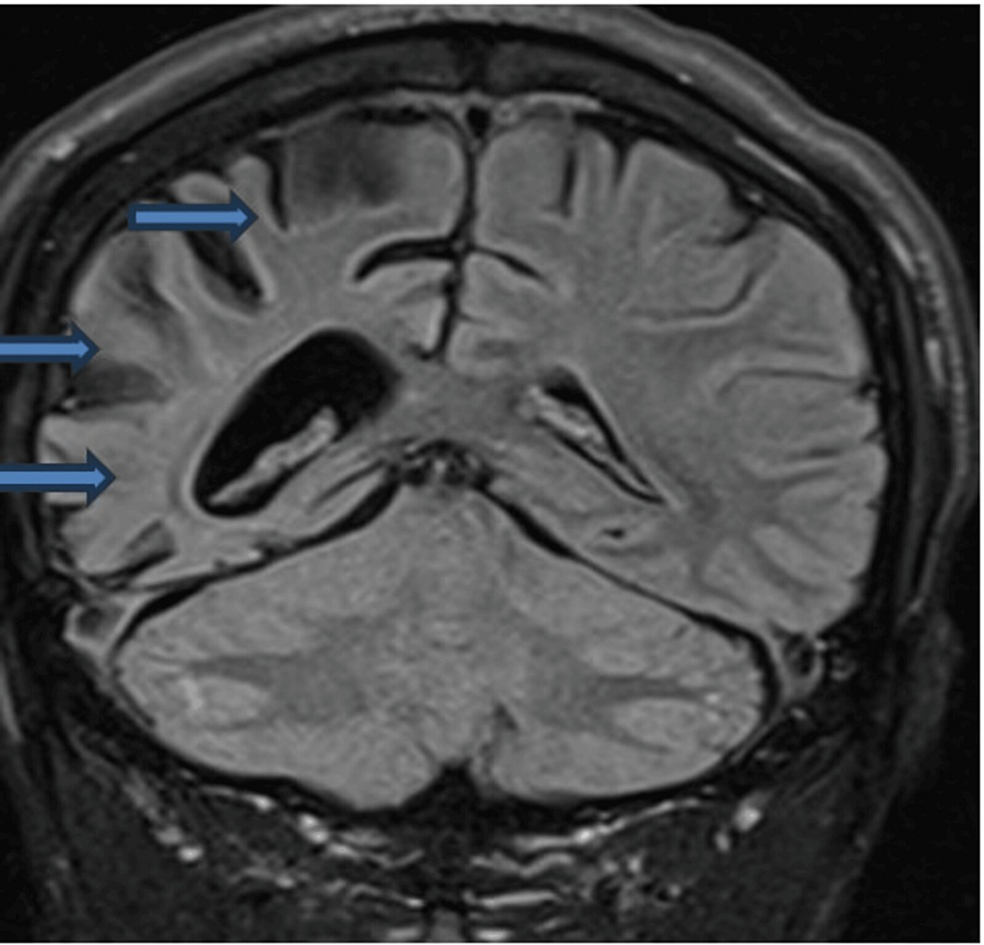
Coronal section of a non-contrast MRI of the head done on the patient at age 33 revealing atrophy of the right cerebral hemisphere.
